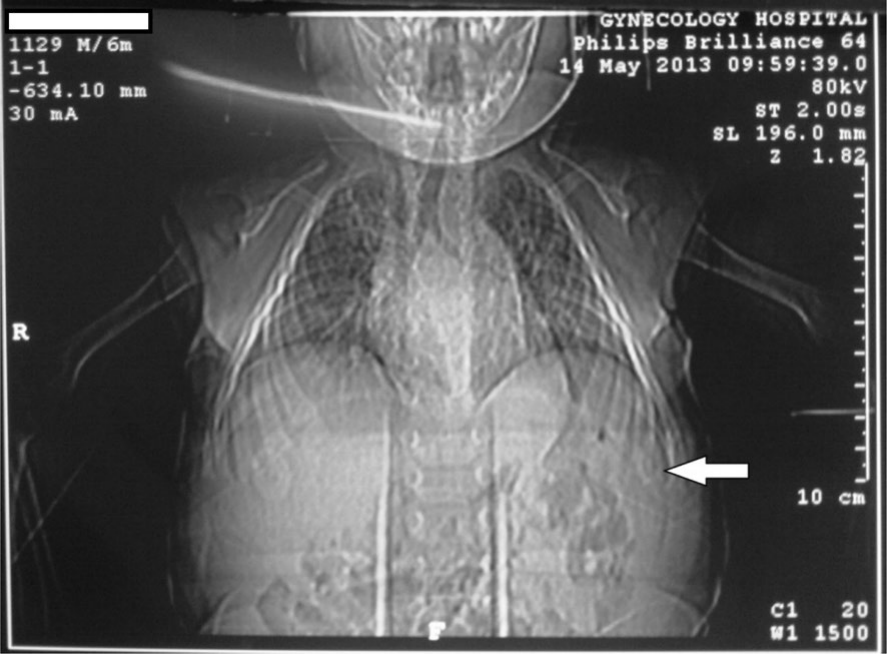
Plain chest radiograph showed an irregular 20 × 10 mm lytic expansile lesion (*arrowed*) involve and covering the anterior angle of the 8th rib.
